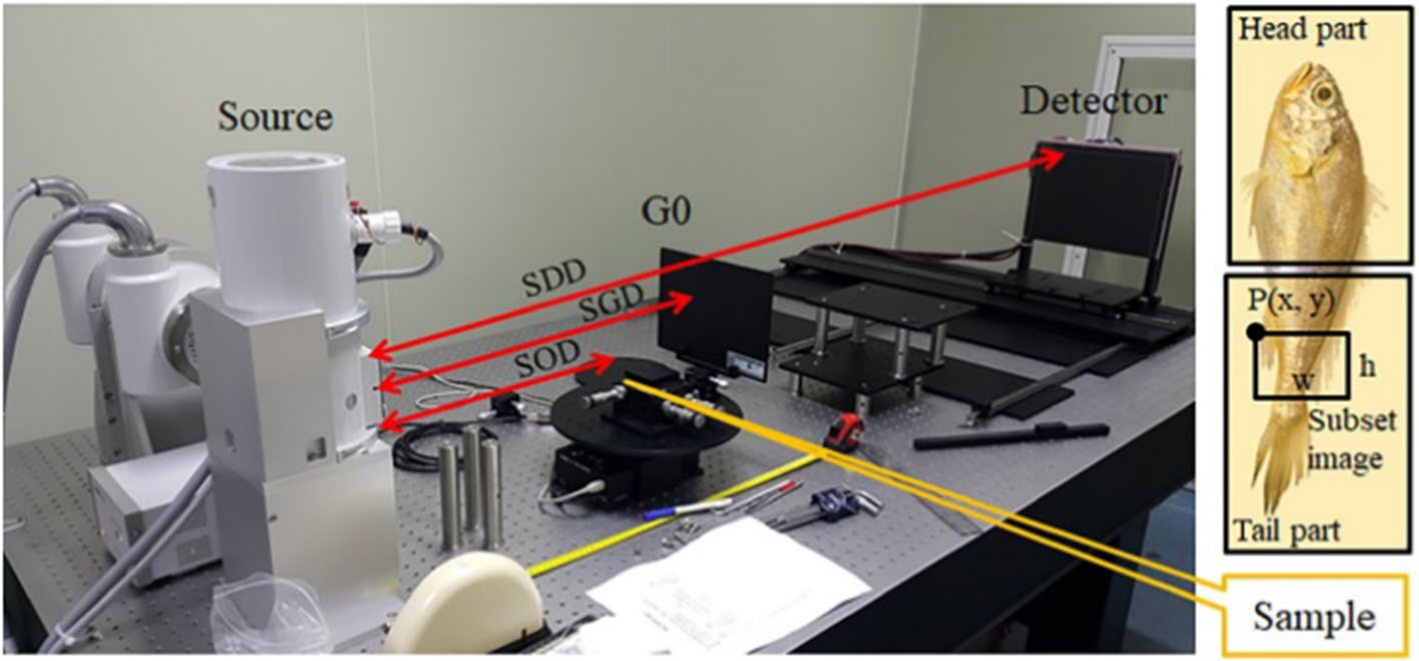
Setup of the equipment used to obtain the raw images. The X-ray tube was operated at 22 kVp and 20 mA, with an exposure time of 630 ms. The focal spot size of the equipment was 100 μm and the grid pitch was 0.118 mm (215 dpi). The CMOS flat-panel detector had a pixel size of 7 µm and dimensions of 3840 × 3072. The source-to-object distance (SOD), source-to-grid distance (SGD), and source-to-detector distance (SDD) were 500, 700, and 1448 mm, respectively
